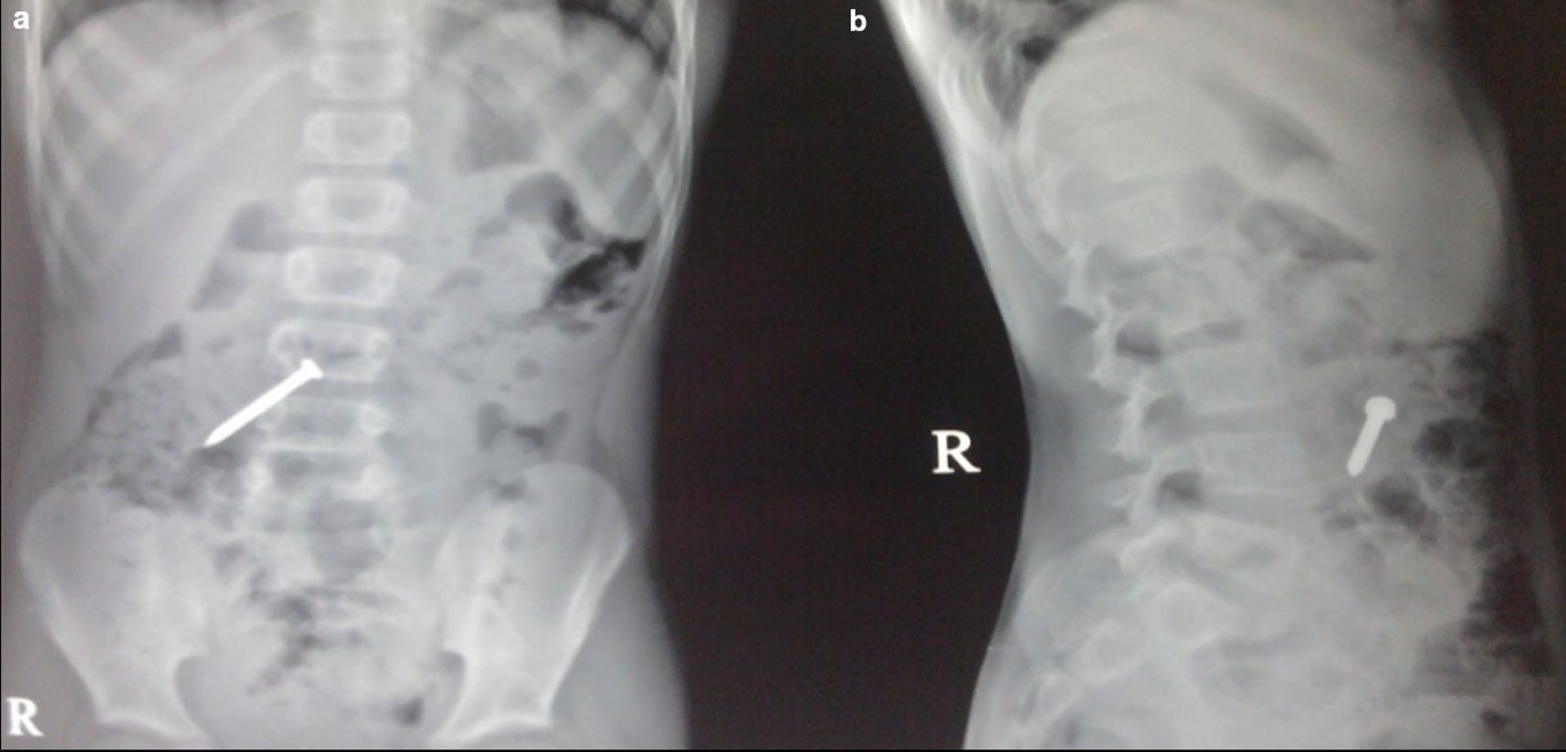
a & b: Radiograph of the abdomen in frontal and lateral views reveals a nail at the level of the L4 and L5 vertebrae in the gastrointestinal tract.
